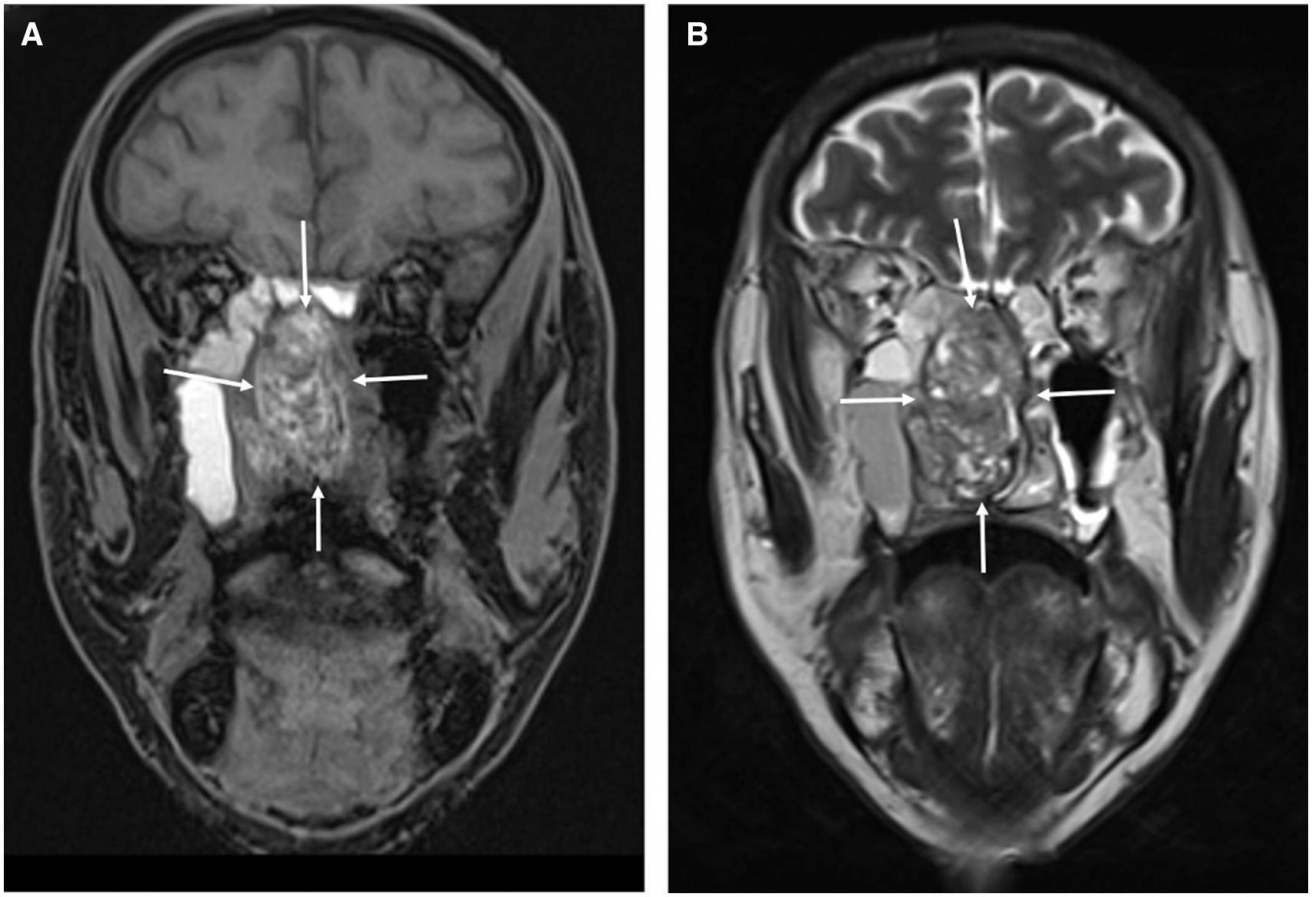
Primary sinonasal melanoma. (A) Coronal fat-saturated pre-contrast T1-weighted MRI and (B) coronal T2-weighted sequence show an expansile heterogeneous nasal cavity lesion (arrows) with foci of intralesional high T1 signal representing melanin/haemorrhage. Foci of high signal and areas of signal void on the T2-weighted sequence (B) are consistent with internal cystic change and old blood products, respectively.
